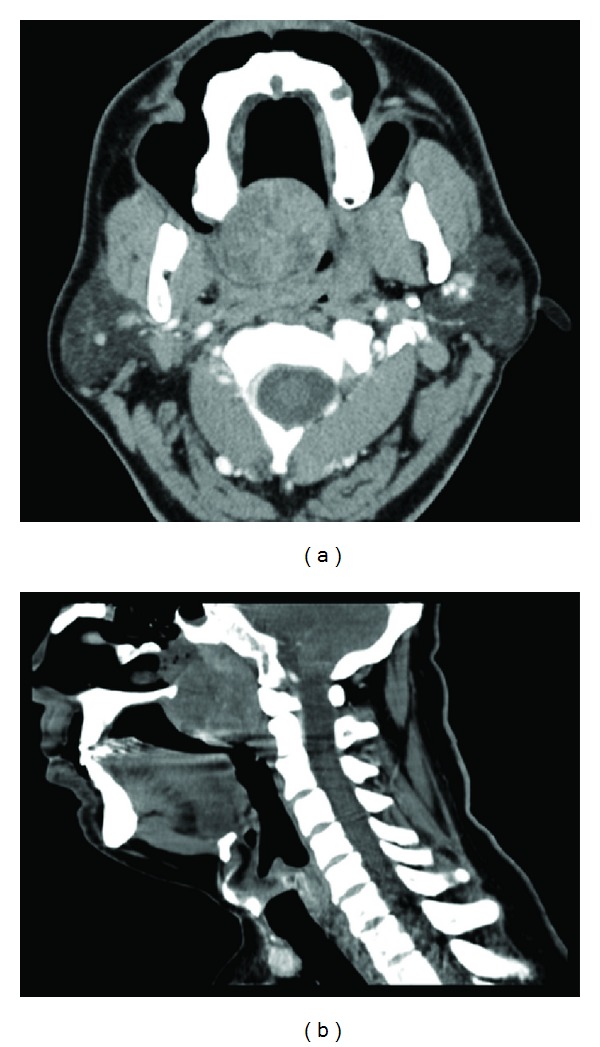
(a) Axial and (b) sagittal contrast-enhanced CT scan demonstrated a well-circumscribed, enhancing solid mass (5 × 3.5 cm) originating from the right posterior portion of the soft palate and enlarging toward the oronasopharyngeal airway space, but the lesion did not infiltrate adjacent fat plans and did not appear to involve the bone.
